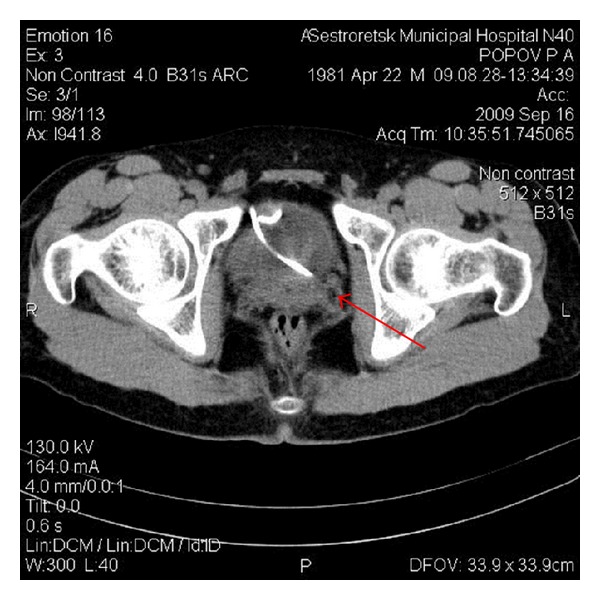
CT showing no stone in the ureter; the area of previous stone location is shown by red arrow.
